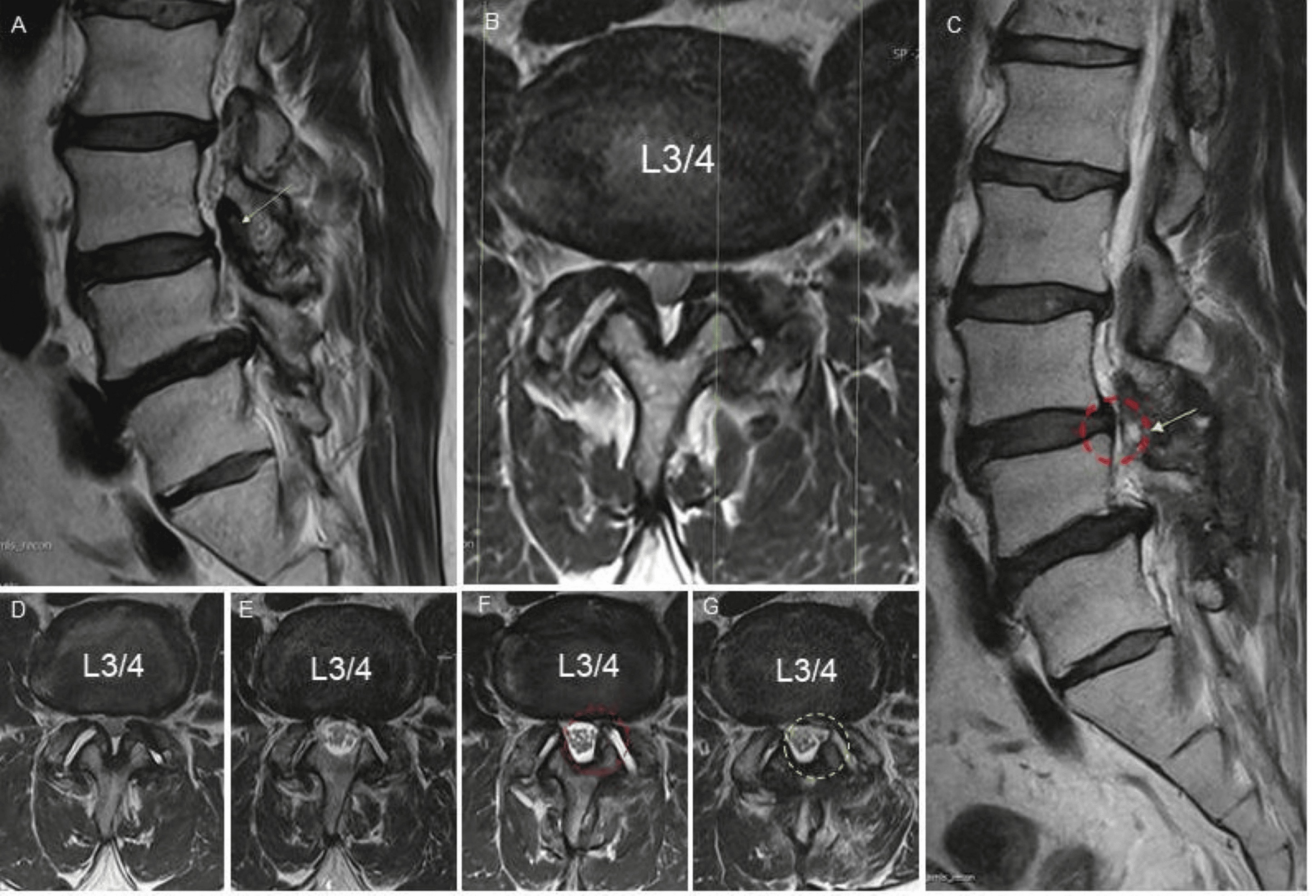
Case 2 MRI Timeline Sagittal and axial T2-weighted MRI preoperative images (L3-4 LSS + DSL Grade 1 and L4-5 foraminal stenosis) (white arrow indicating the L3-4 level) (A) with facet degeneration (B). Sagittal view of the FC lesion location (red circle) after seven months period follow-up (C). Progression timeline axial T2-weighted MRIs: Preoperative (D), Post-ILP (E), FC development (F), and final decompressed lateral recess state (white circle) (G). LSS: lumbar spinal stenosis, DSL: degenerative spondylolisthesis, ILP: interspinous ligamentoplasty, FC: facet cyst.
